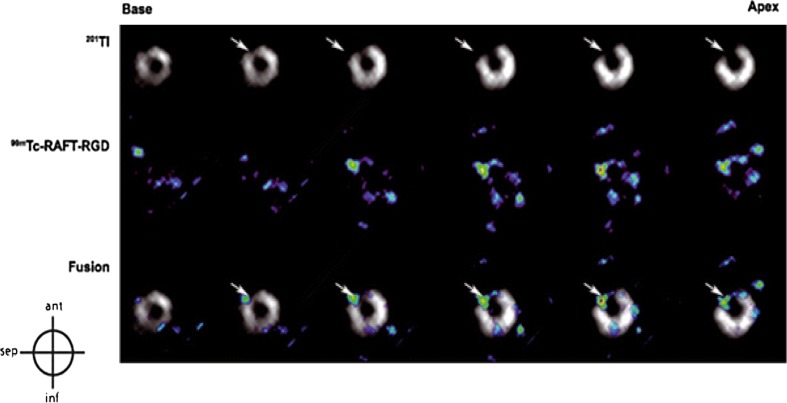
Myocardial short-axis images from base to apex with ^201^Tl perfusion SPECT, ^99m^Tc-RAFT-RGD SPECT scan and fused images. The arrows highlight areas of infarct (figure adapted from [21])
